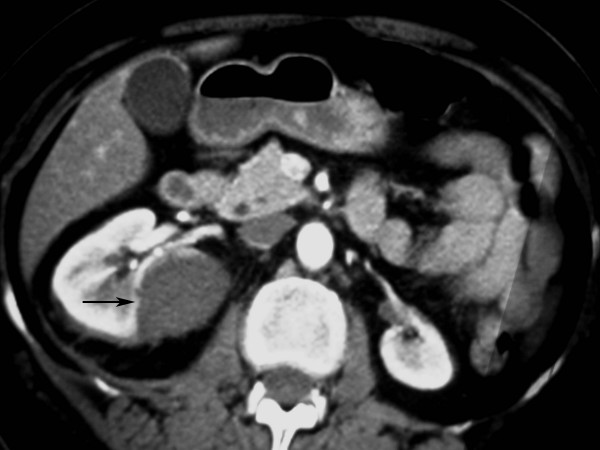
Follow-up CT scan at 24 hours showed the RF ablated area with no stricture of the renal pelvis and necrosis of tumour.
